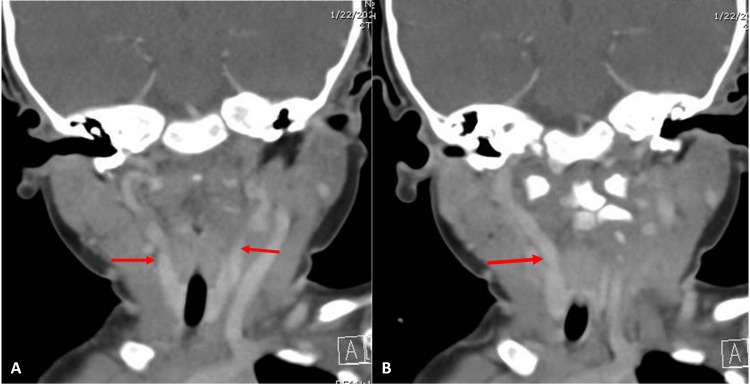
CT scan demonstrating medialized carotid arteries (red arrows) Note that there was likely movement artifact as the CT scan was obtained without sedation, and there was likely movement.
